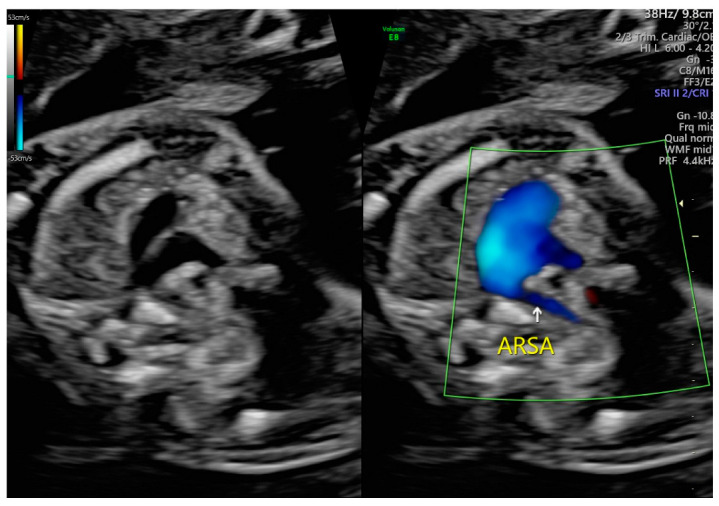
Color Doppler ultrasound images showing a fetal aberrant right subclavian artery at 22 weeks of gestation.
